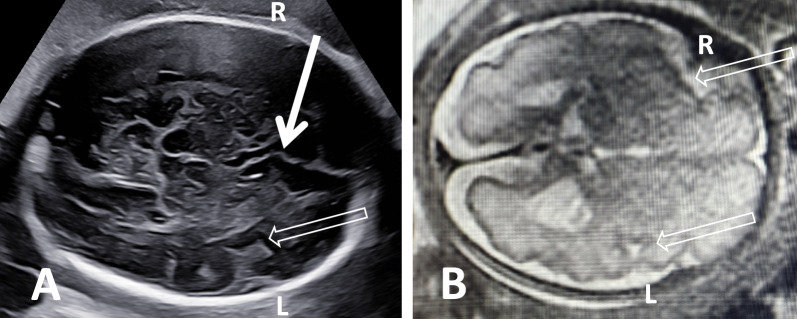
Patient 30, the 29.3-week-old fetus of a 30-year-old woman, had bilateral abnormal SF. A Axial plane showing an abnormal SF (hollow arrow) in the distal hemisphere; note the distortion of the anterior midline (arrow). B MR image showing the asymmetric sulci on the lateral surface of the brain; note the narrow-plateau SF in the left hemisphere and the nonplateau SF in the right hemisphere (hollow arrows). WES-Trio revealed a variant in the *TUBB3* gene, which may cause complex cortical dysplasia with other brain malformations
